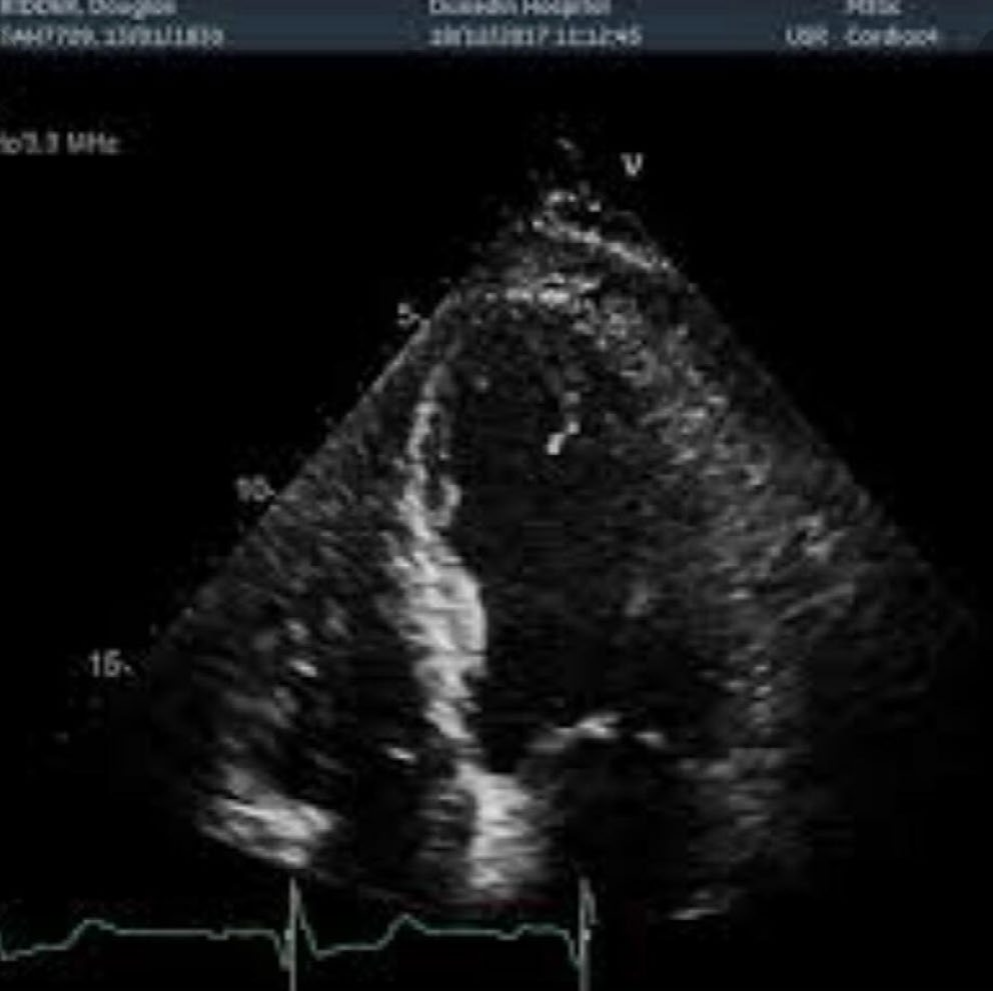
Transthoracic echocardiography revealed anterior wall and apical segments akinesia consistent with anterior MI
